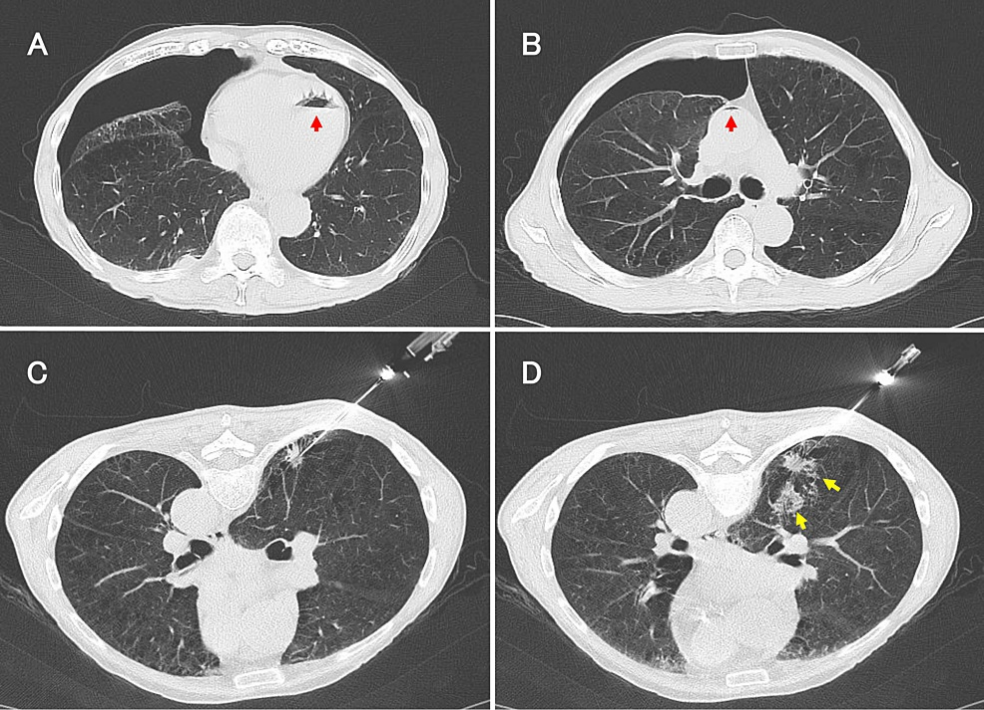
Chest CT images during CT-guided lung needle biopsy in case 1. Axial chest computed tomography images post lung needle biopsy, showing air within the left ventricle (A) and aorta (B). At the beginning of the examination, there was no bleeding around the nodule (C); however, bleeding occurred during the examination (D).
